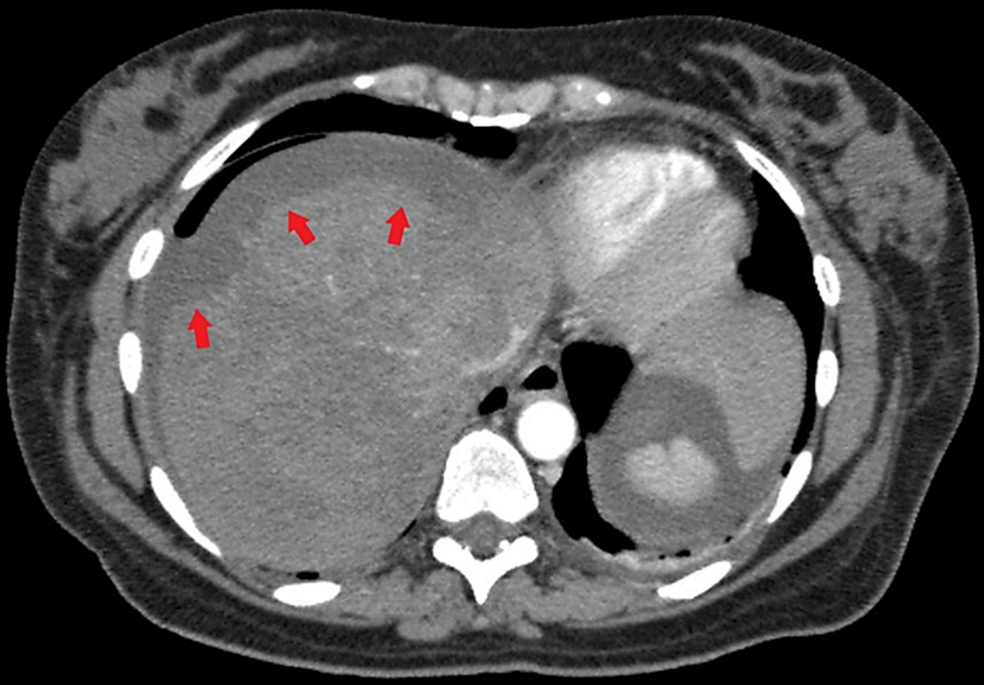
Abdominal CT-Scan in the transverse plane showing a large subcapsular liver infiltrate consistent with a hematoma. The capsule is intact.
